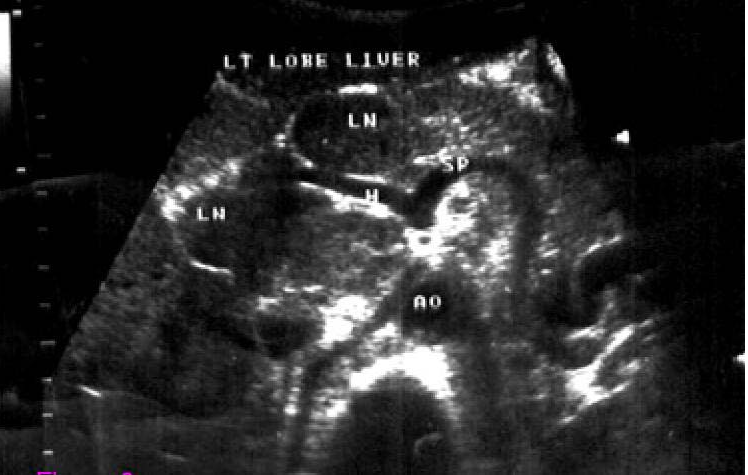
Transverse sonogram showing extensive periportal adenopathy in a HIV+ patient with non-Hodgkin lymphoma. AO-aorta, H-hepatic artery, SP-splenic artery, LN-lymph node.
